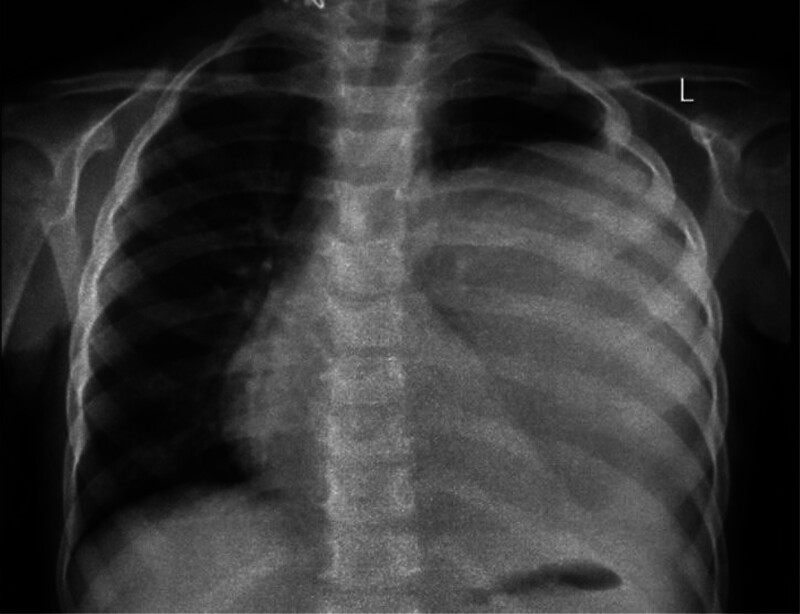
Chest X-ray (PA view) demonstrates a large lesion occupying most of the left hemithorax, with a noticeable mediastinal shift to the contralateral side. There is also depression of the ipsilateral diaphragm, suggesting significant space-occupying pathology in the left lung.
